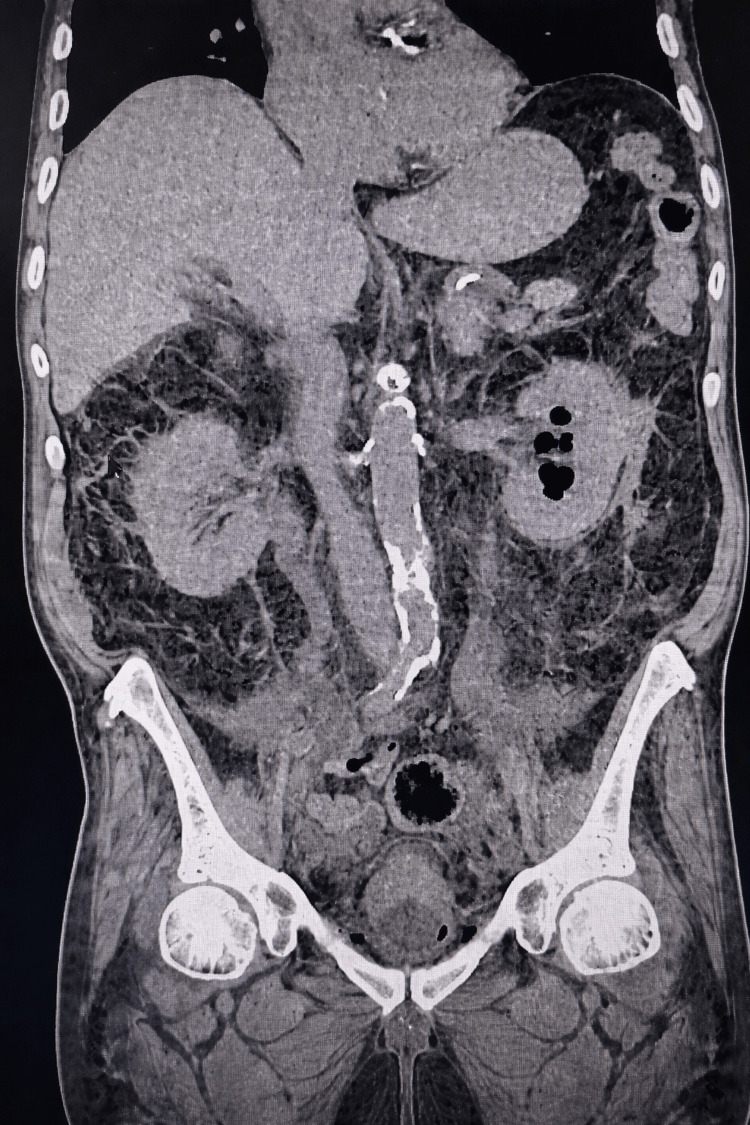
Emphysematous pyelitis and cystitis on CT abdomen and pelvis Contrast-enhanced CT of the abdomen and pelvis, demonstrating findings consistent with emphysematous pyelitis and cystitis, characterized by the presence of gas within the left renal collecting system and along the bladder wall. No evidence of bladder or ureteral perforation is identified CT: computed tomography
